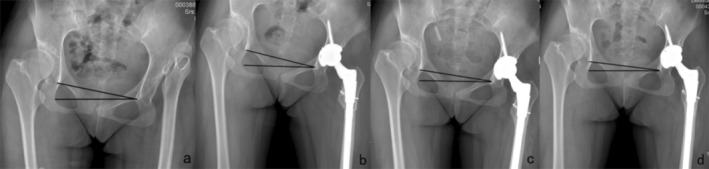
(a–d) The patient, female, 22 years old, with a pre‐operative diagnosis of DDH Crowe type IV and combined type IB infrapelvic obliquity. DAA‐THA was performed successfully. (a) The pre‐operative radiograph shows significant pelvic obliquity to the affected side. (b) The pelvic obliquity is mildly improved after 1 month of DAA‐THA compared to the pre‐operative radiograph. (c) Six months postoperative radiographs showed a significant improvement in pelvic obliquity of the patient. (d) At the last follow‐up, the degree of pelvic obliquity on radiographs continued to be improved, but some pelvic obliquity remained.
